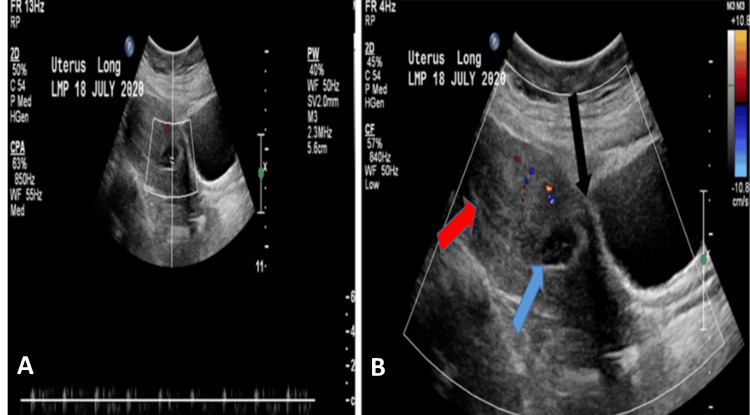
Longitudinal transabdominal ultrasound demonstrating the characteristic features of cesarean scar ectopic pregnancy Low-lying gestational sac (blue arrow) with positive cardiac activity, which is implanted in the area of the CS scar with thinning of the anterior myometrium between the gestational sac and uterine serosa (black arrow). The endometrium is empty (red arrow). A: characteristic features of CSEP; B: details about gestational sac, cardiac activity, and endometrium
